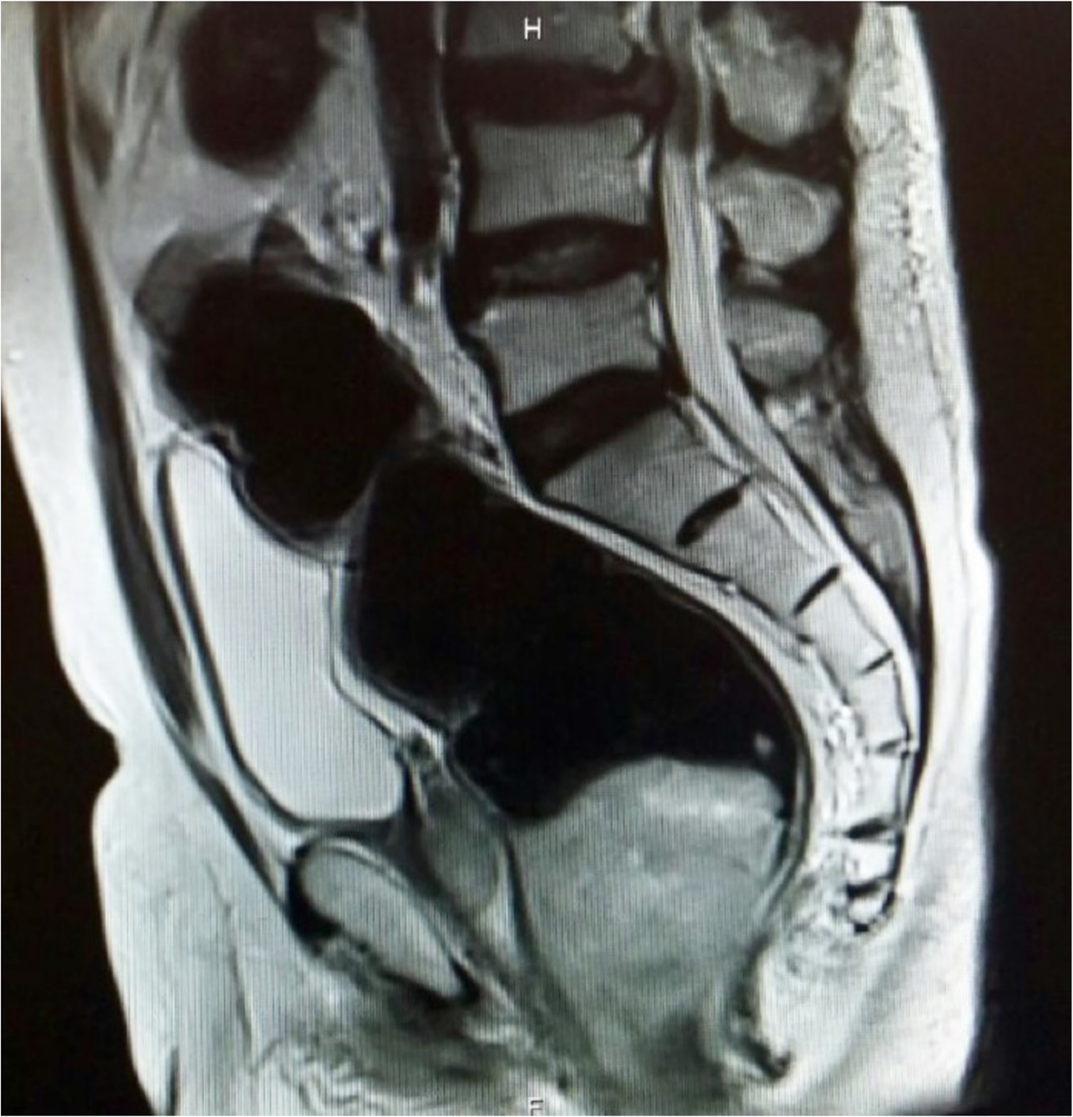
Sagittal T2-weighted magnetic resonance image shows hyperintense areas of the distal rectum mass, showing no invasion to bladder or prostate
